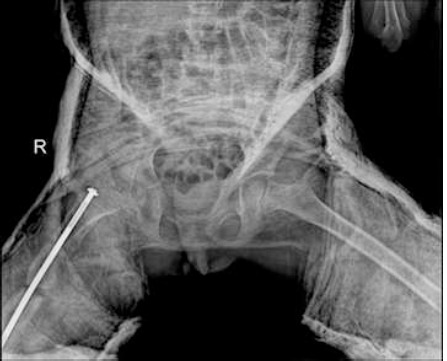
anteroposterior hip X-ray—after hip reduction under anaesthetic in the cast
